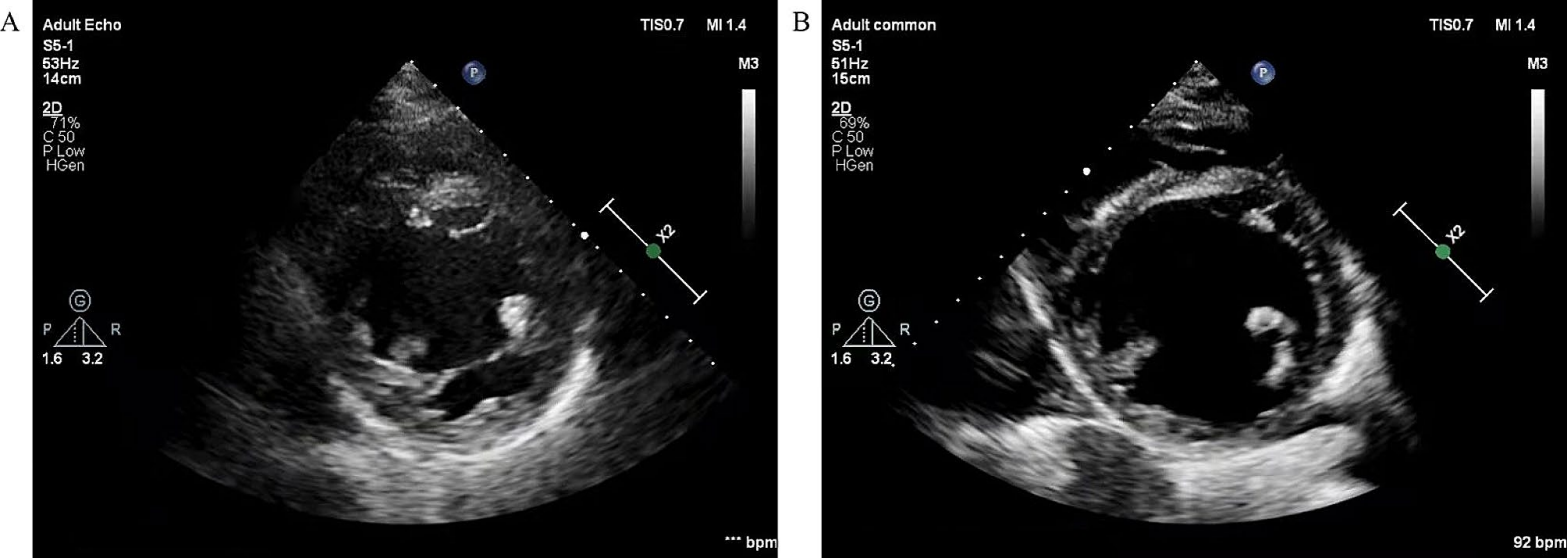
Echocardiographic imaging from Parasternal left ventricular short axis view. (A) Echocardiographic imaging at ICU admission. (B) Echocardiographic imaging at 72 h after the Sivelestat treatment
